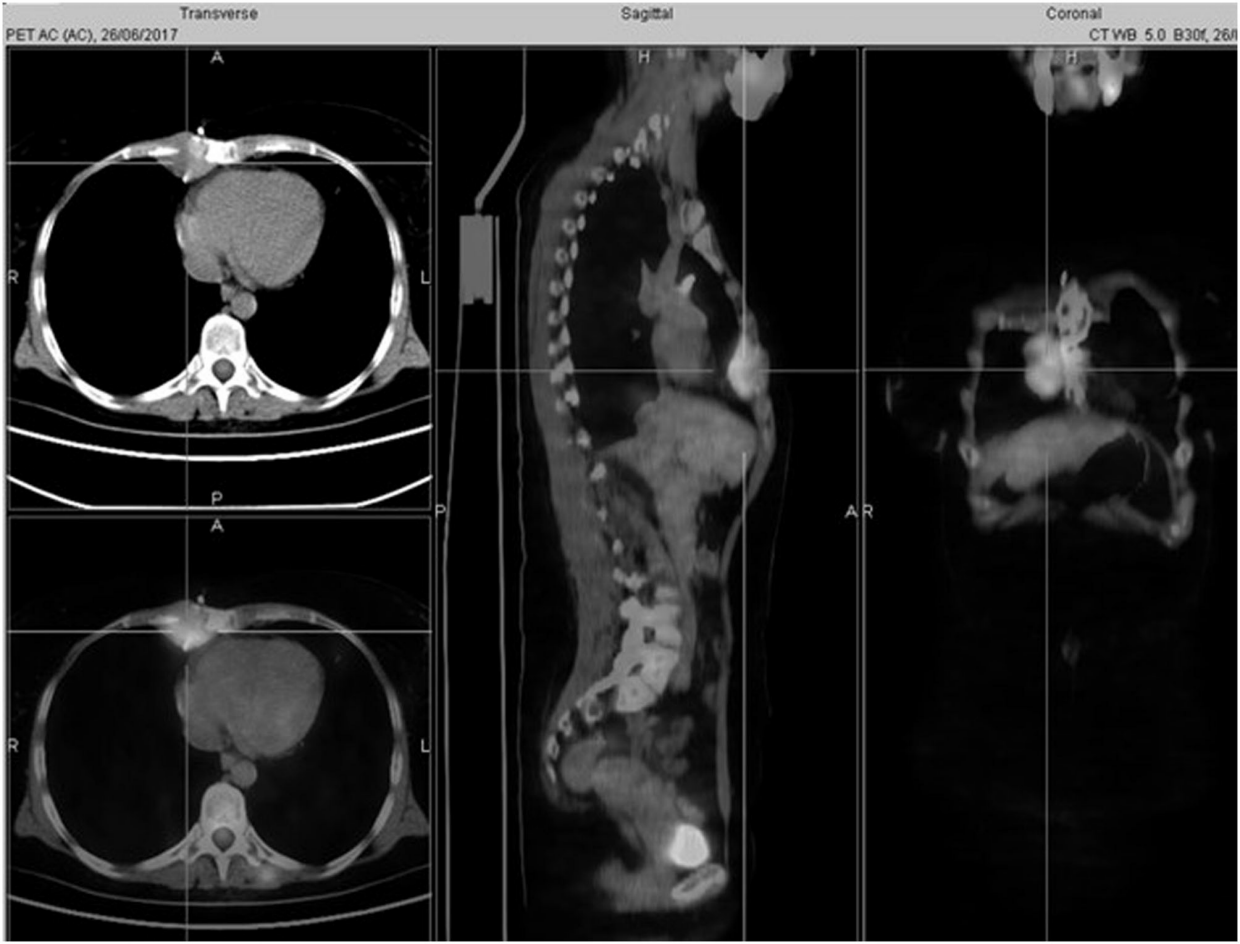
Preoperative PET/CT displayed a right paramediastinal mass infiltrating the sternal body.
